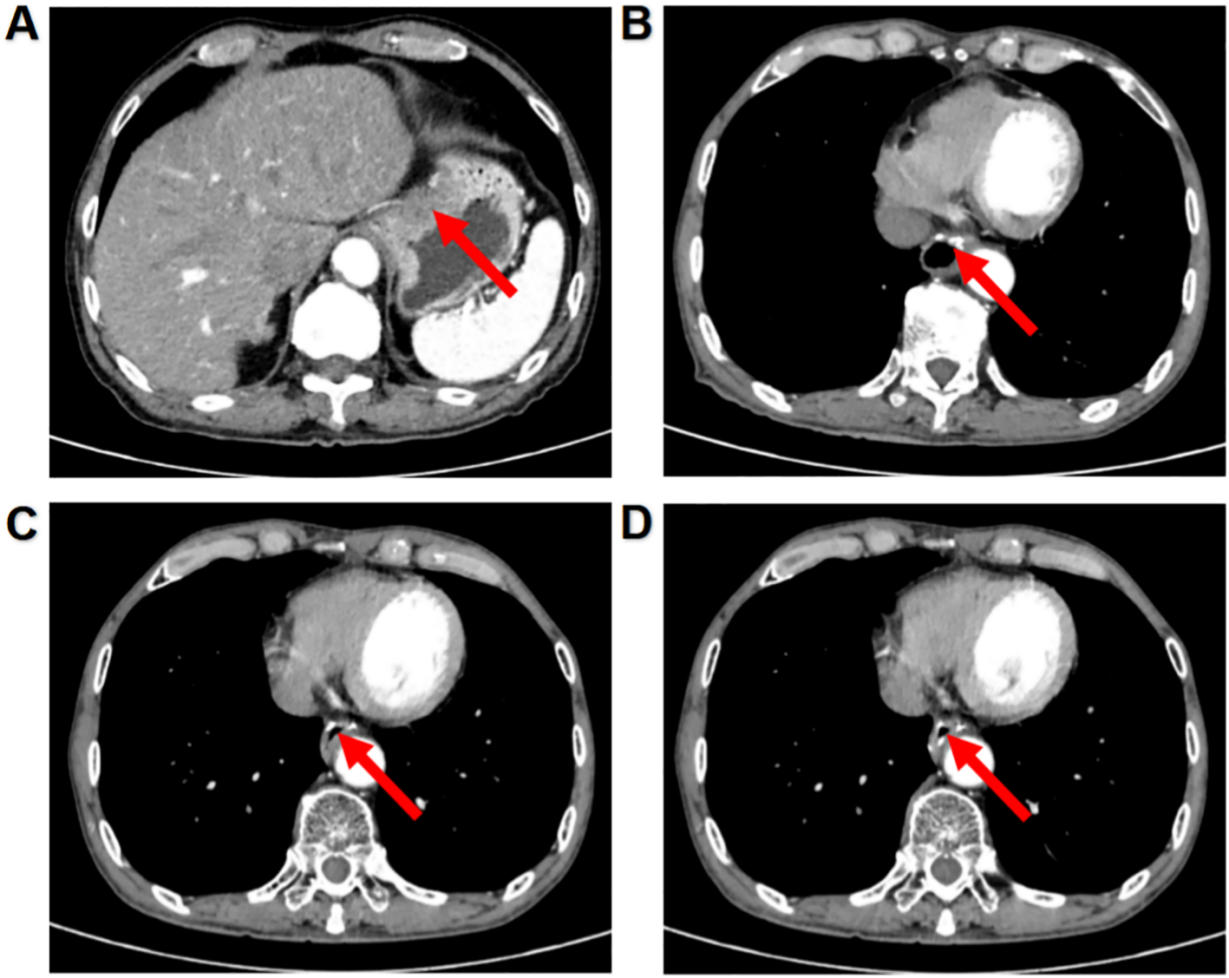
Imaging evaluation of the patient from diagnosis onward. (A) Abdominal contrast-enhanced CT image on March 30, 2023, revealing a mass located at the gastric fundus–lesser curvature junction. (B) Non-contrast abdominal CT scan on July 5, 2023, performed during the episode of immune-mediated subacute liver failure. (C) Contrast-enhanced abdominal CT on November 9, 2024, showing postoperative changes following resection of the gastric mass, with no evidence of recurrence and stable disease. (D) Contrast-enhanced abdominal CT on June 5, 2025, demonstrating continued stability without tumor recurrence.
